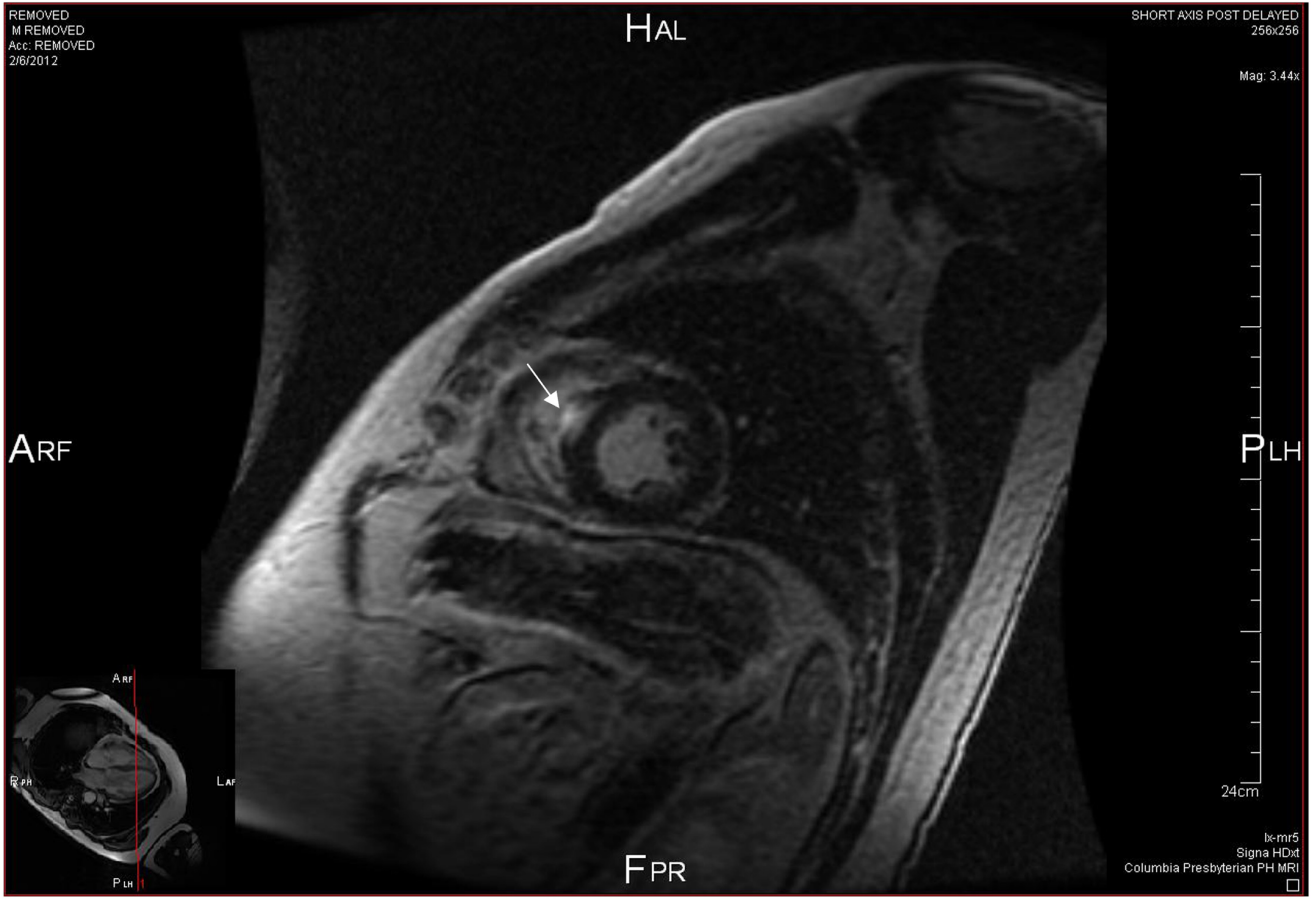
Cardiac MRI sagittal view at mid ventricle level showing focal late gadolinium enhancement (white arrow) in the interventricular septum.
